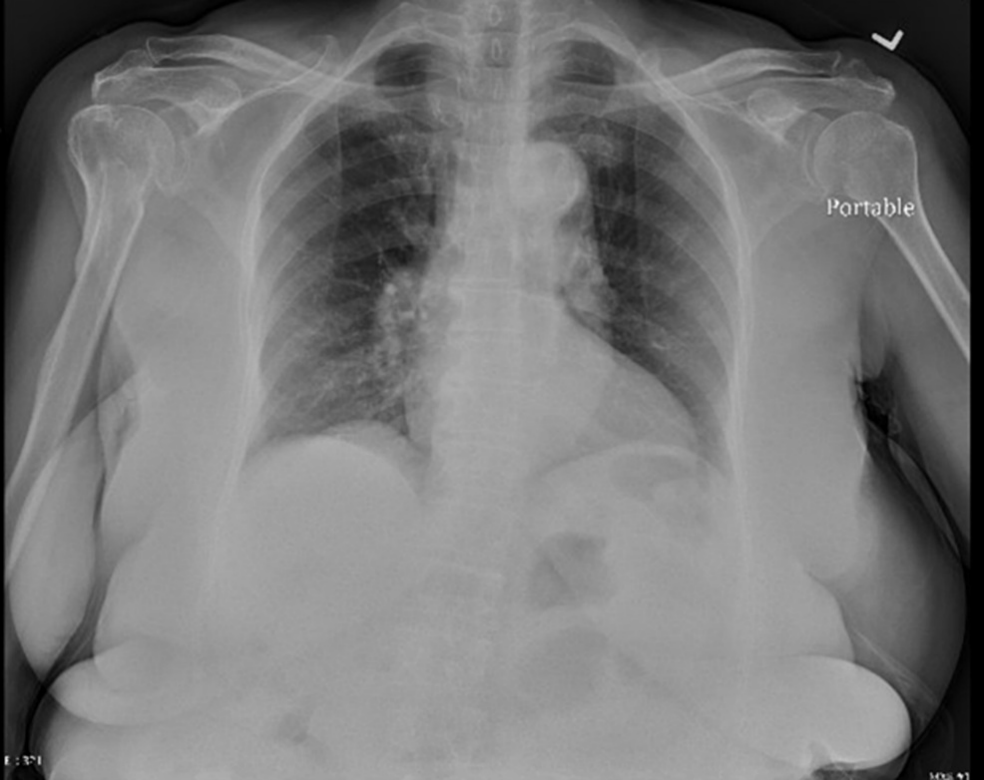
Chest X-ray of the patient on admission shows no acute findings
